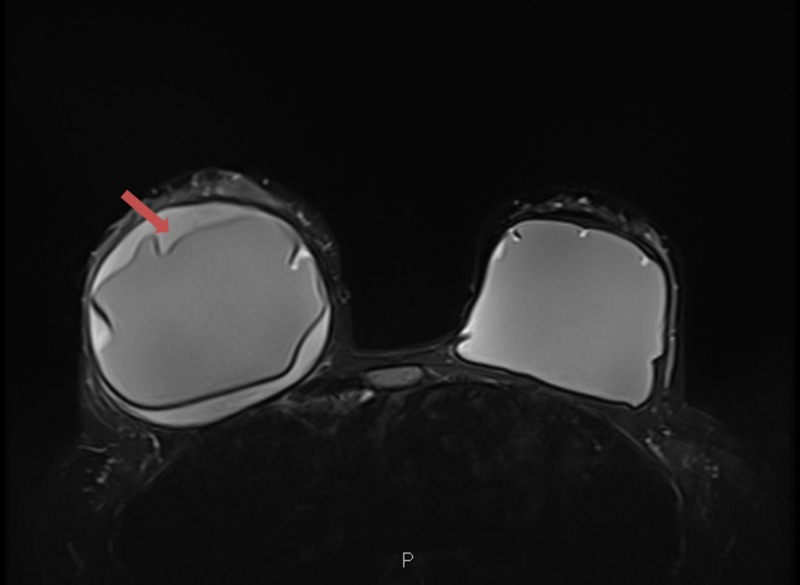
Bilateral breast magnetic resonance imaging (MRI). There is a large fluid collection, indicated by the red arrow, surrounding the intact right silicone implant. The fluid is interposed between the fibrous capsule and the implant.
